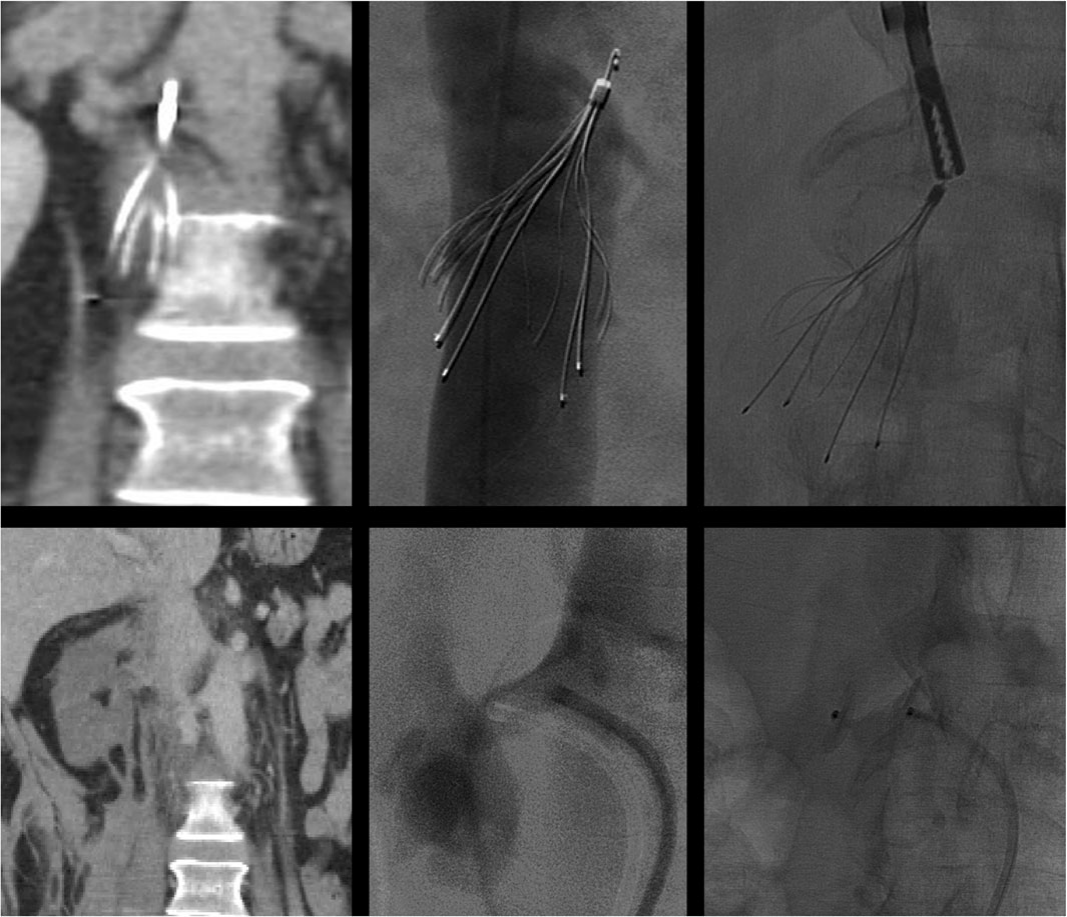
This patient had undergone placement of a Cook Celect filter (Cook, Bloomington, IN) 24 weeks prior to presentation for filter retrieval. Pre-procedure CT showed the right hook of the IVC filter to be protruding into the right renal artery. Cavagram showed an angulated filter with an embedded hook and therefore bronchial forceps were used. After technically sucessful filter retrieval, the patient developed hypotension and right sided flank pain. CT showed a right sided retroperitoneal hemorrhage and an infarcted right kidney. Angiogram was done demonstrating a fistula between the right renal artery and IVC and the renal artery was embolized with a 12 mm Amplatzer Vascular plug. In this case, with pre-procedure CT evidence of the hook penetrating into the renal artery, obtaining arterial access could have been done to allow imaging and potential treatment of any renal arterial disruption during retrieval
